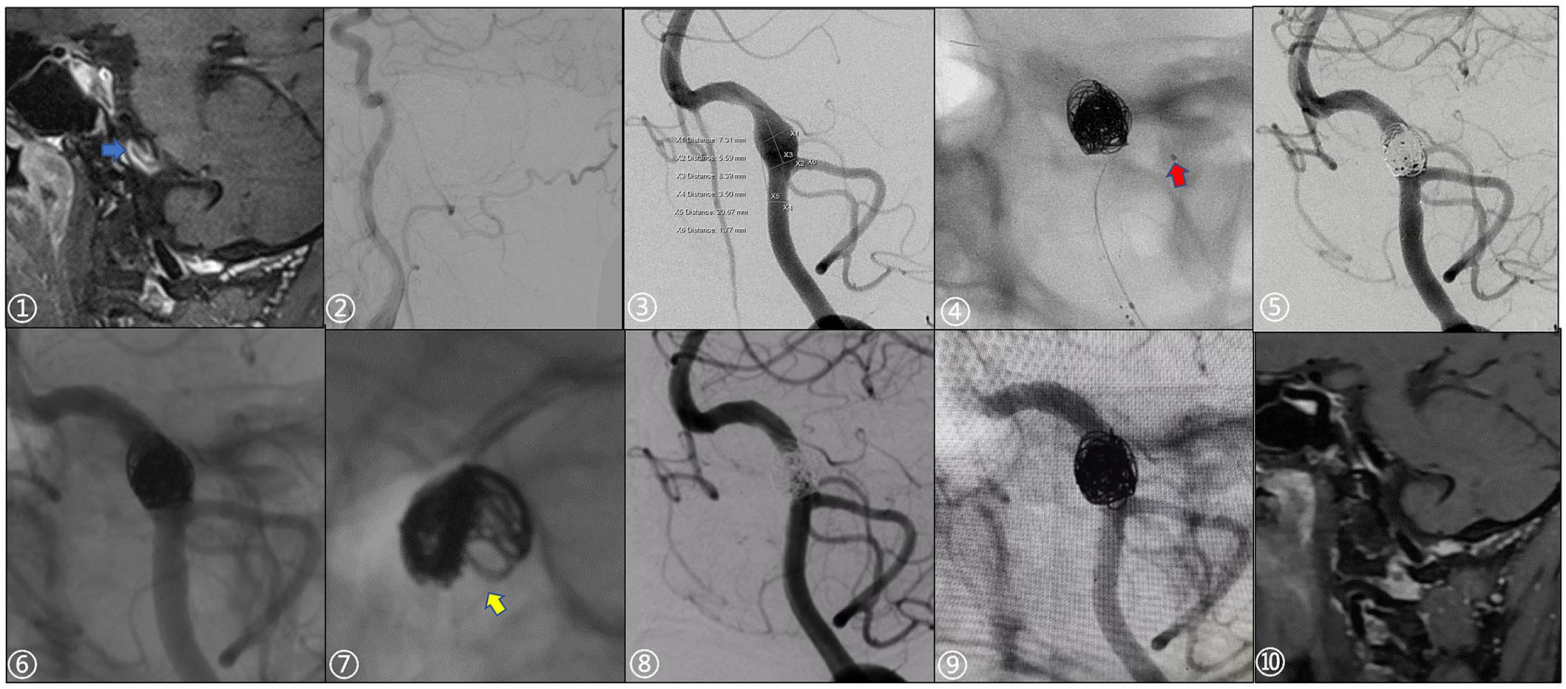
A 49-year-old male patient presented with a sudden severe headache once 2 months prior. ① Preoperative high-resolution MRI showed significant enhancement of the vessel wall with the intraluminal slow flow (blue arrow). ② Right vertebral artery dysplasia. ③ Preoperative measurement of aneurysm and parent artery, aneurysm size: 7.31 x 8.39 mm. ④ Echelon-10 microcatheter (red arrow) was used to protect the posterior inferior cerebellar artery during operation. ⑤, ⑥ Stents were post-deployed, Immediate postoperative angiography and non-subtraction images showed slight stagnation in the aneurysm sac (Raymond IIIa; Microplex-10 coils: two 8 x 30 cm and one 7 x 30 cm; LVIS stents: 4.5 x 20 mm, 4.5 x 15 mm). ⑦ The distribution of the coils along the wall was not well-uniform. ⑧, ⑨ 12-month follow-up showed that there was no recurrence of the aneurysm (Raymond I) and the parent artery was patent. ⑩ 12-month follow-up with HR-MRI, the vortex in the sac disappeared, while the aneurysm wall was still partially enhanced.
